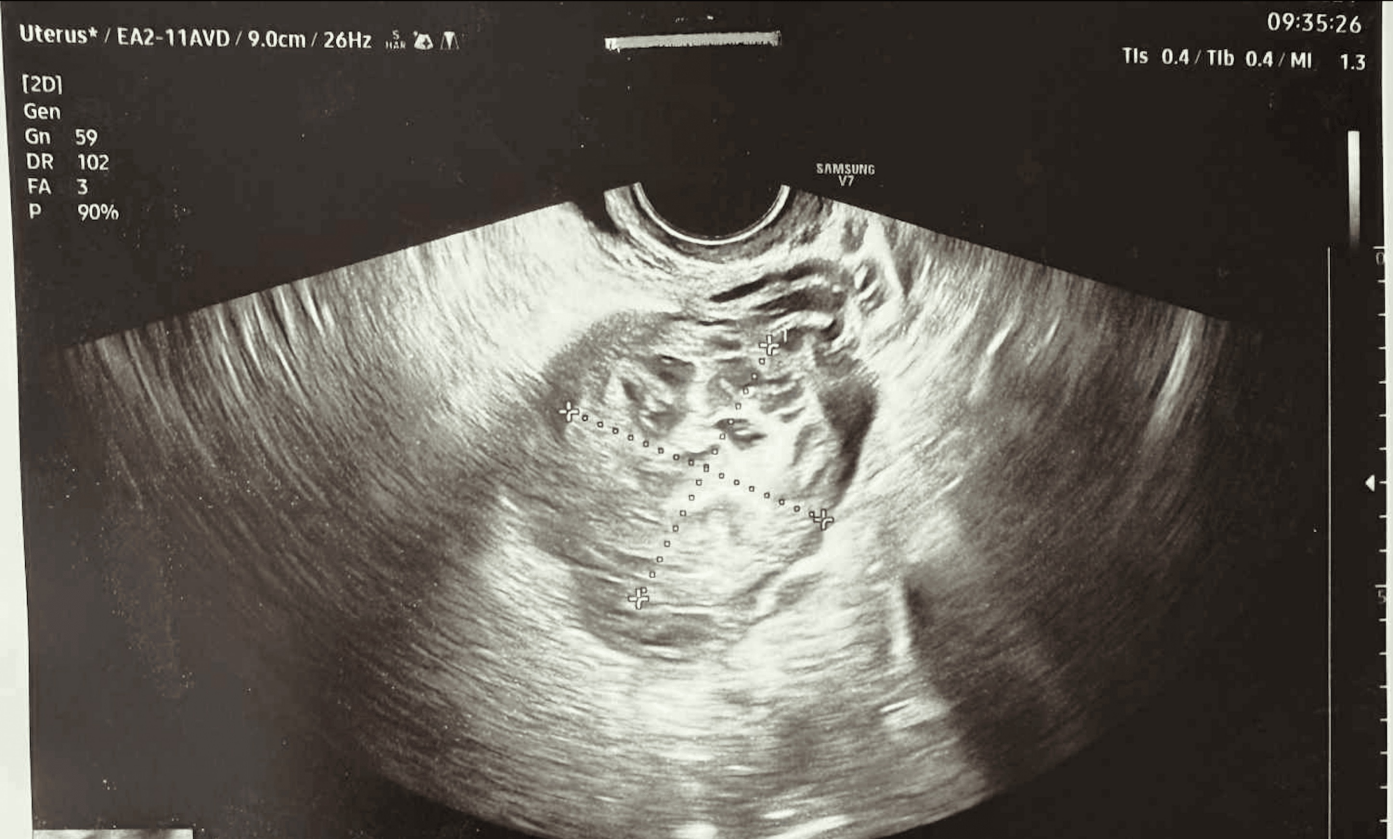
Progression of the lesion on ultrasound
